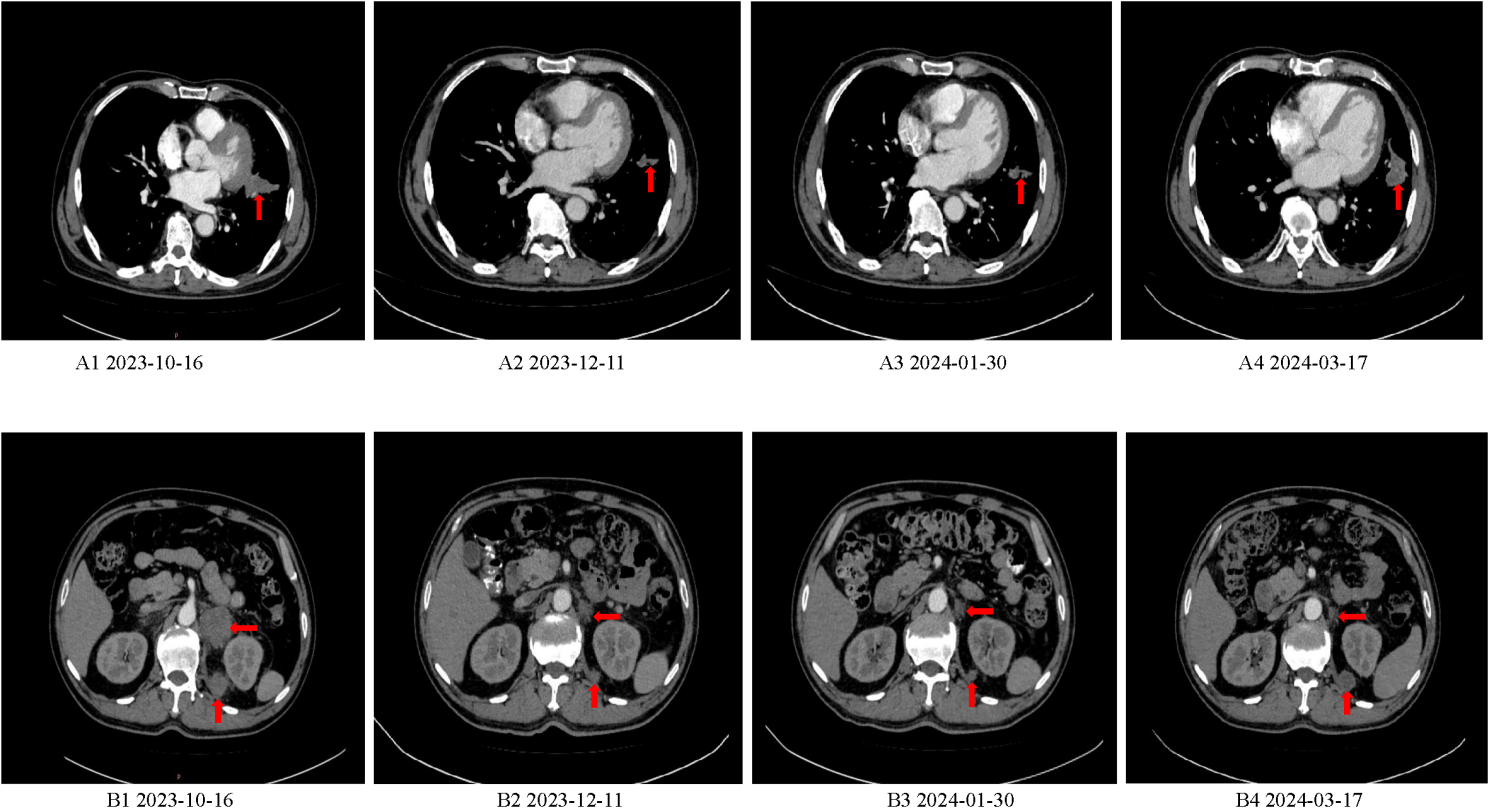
Baseline CT and CT after efficacy evaluation. (A1, B1) CT scan showed irregular strip soft tissue mass in the left hilar and upper lobe of the left lung, with a maximum cross-section of 4.7×3.0cm, left adrenal and left retrorenal masses and abdominal lymph nodes were considered to have metastasis. (A2, B2) CT scan showed that the soft tissue mass of left hilar and upper left lobe was reduced, and the metastatic lesions of left adrenal gland and posterior left kidney were smaller than before. (A3, B3) CT scan showed that the lesions of the left hilar - upper lobe of the left lung, double adrenal gland and internal posterior metastatic lesions of the left kidney continued to shrink compared with Dec 11, 2023. (A4, B4) CT scan showed that the soft tissue lesions in the left upper lobe of the lung and the metastatic lesions of posterior left kidney were enlarged again.
